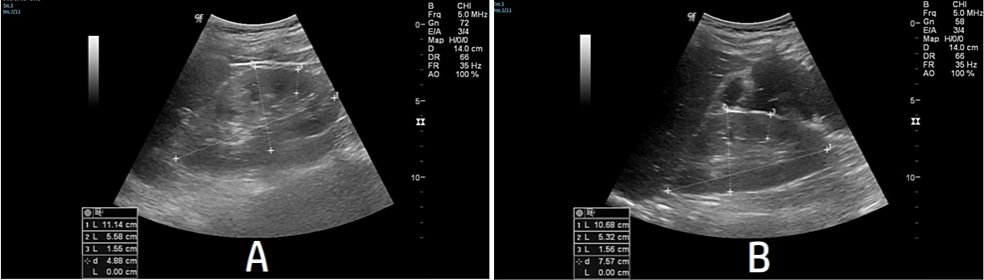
Ultrasound Images: A. Left kidney B. Right kidney
